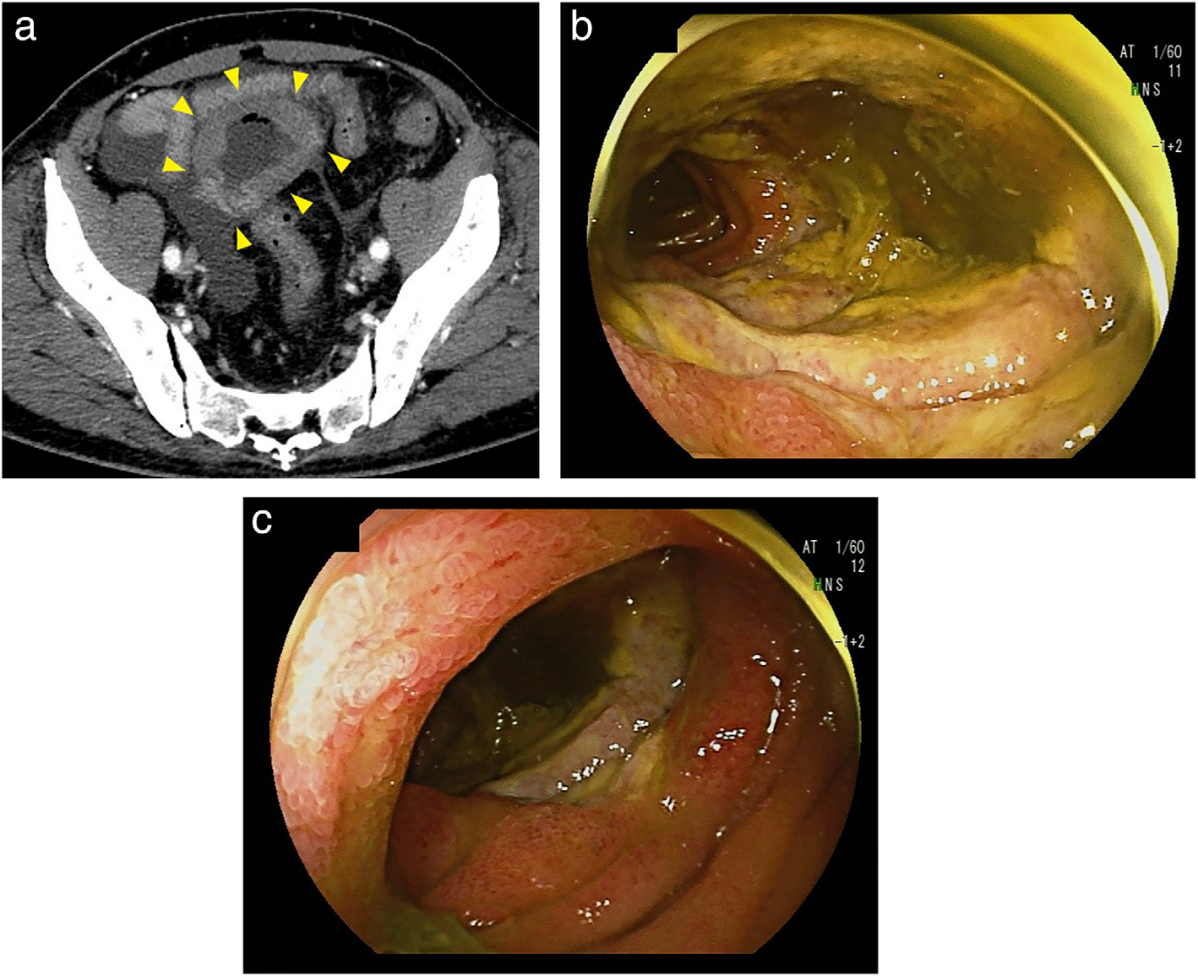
(a) Contrast‐enhanced abdominal CT showing circumferential wall thickening and luminal dilatation in the jejunum (arrowheads). (b) Endoscopic view of the jejunum showing a deep, nearly circumferential ulcer. The ulcer base was covered with a thick slough and (c) the ulcer was surrounded by a granular membrane with swollen villi.
